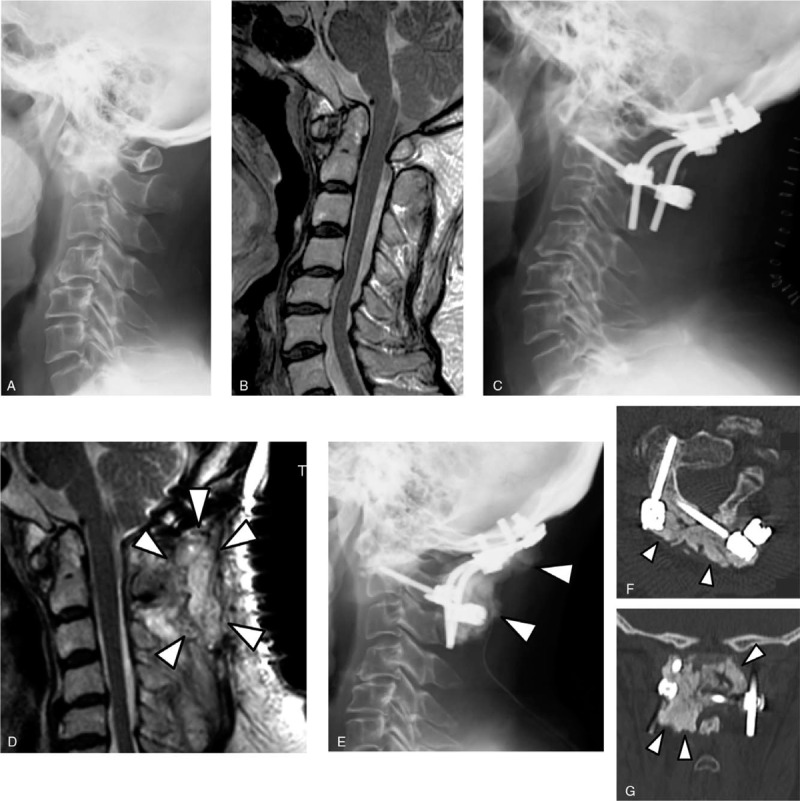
Case 2: (A) Preoperative lateral view radiograph. (B) Preoperative mid-sagittal T2-weighted MRI. (C) Postoperative lateral view radiograph. (D) Mid-sagittal T2-weighted MRI taken after the occurrence of deep SSI, revealing abscess formation in the subfascial space around the spinal instrumentation (*arrowhead*s). (E) Lateral view radiograph recorded following the procedure for the treatment of deep SSI. ALBC covering metal implants is shown (*arrowhead*s). (F) Axial CT image and (G) coronal CT image following the procedure for the treatment of deep SSI. The images show that ALBC embedded metal implants occupy the dead space in the operated wound (*arrowhead*s). ALBC = antibiotic-loaded bone cement, CT = computed tomography, MRI = magnetic resonance imaging, SSI = surgical site infection.
